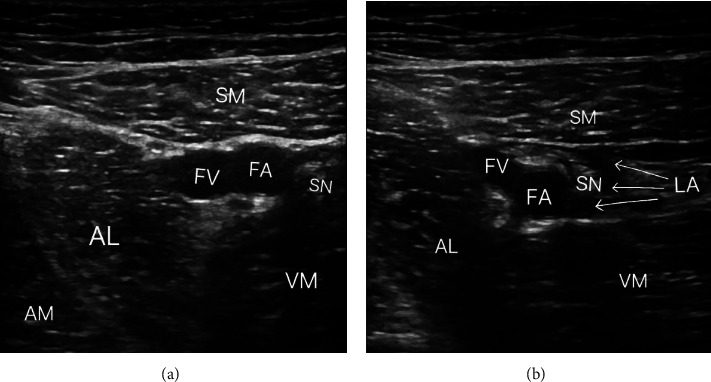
Ultrasound images (a) before and (b) after ACB. Abbreviations: SM, sartorius muscle; AL, adductor longus; AM, adductor magnus; VM, vastus medialis; FA, femoral artery; FV, femoral vein; SN, saphenous nerve; LA, local anesthetic.
